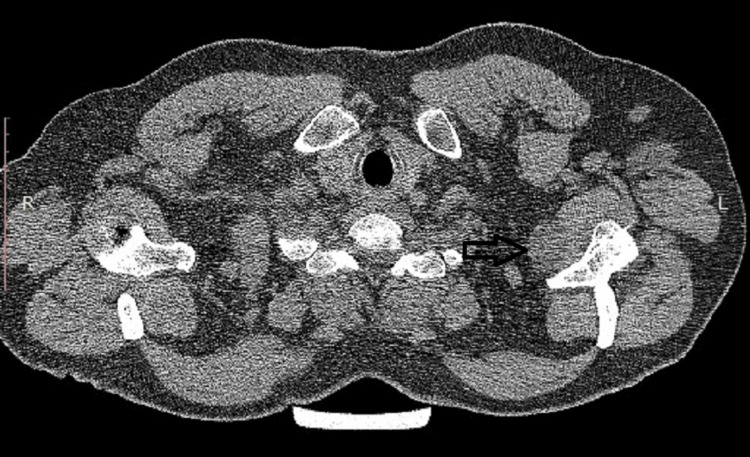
CT image of the cyst as it descends further into the soft tissues
